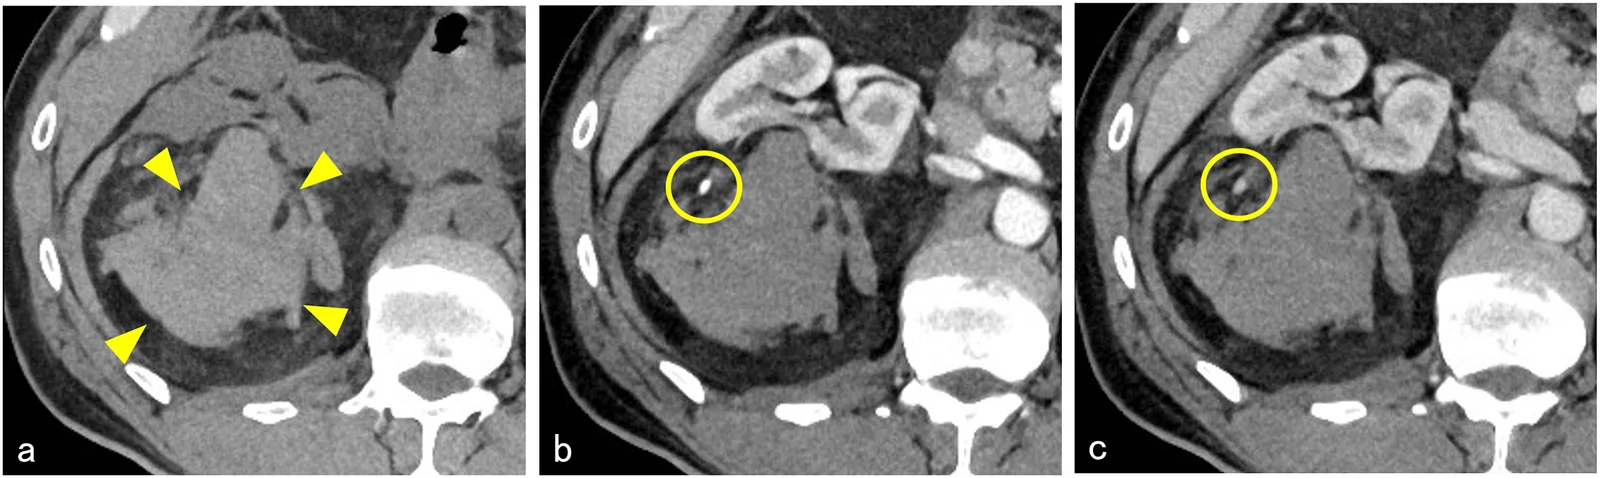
Initial CT findings. (a) Unenhanced CT image demonstrating hematomas associated with rupture of an angiomyolipoma (AML) in the dorsal aspect of the right kidney (arrowheads). (b) Arterial-phase contrast-enhanced CT image demonstrating an enhancing nodular lesion along the ventral aspect of the intratumoral hematoma, suspicious for a pseudoaneurysm and likely arising from the ventral branch of the right renal artery (yellow circle). (c) Equilibrium-phase contrast-enhanced CT image demonstrating persistent enhancement of the pseudoaneurysm along the ventral aspect of the intratumoral hematoma (yellow circle).
